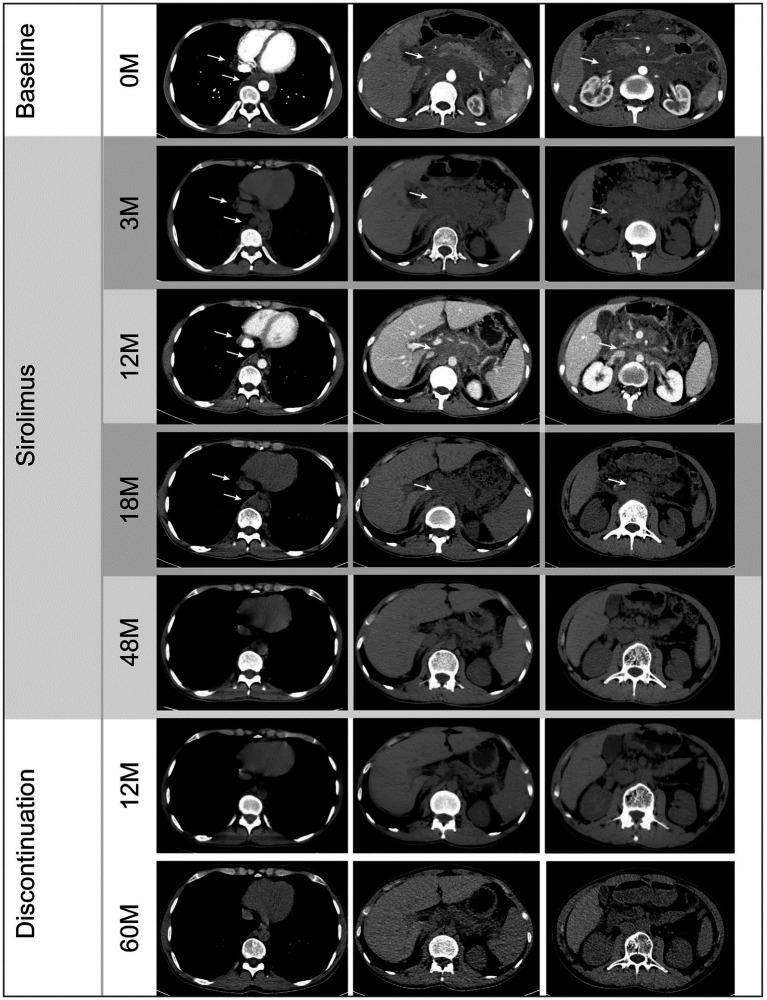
Longitudinal radiologic changes during treatment and follow-up in a patient with kaposiform lymphangiomatosis (KLA). Imaging timepoints include baseline (prior to treatment), sirolimus treatment (Tx) at 3, 12, 18, and 48 months, and post-treatment follow-up (D/C) at 12 and 60 months. “M” denotes months. Baseline CT revealed diffuse soft tissue infiltration in the mediastinum, retroperitoneum, and para-aortic regions. Arrows indicate representative lesions at each timepoint. Lesions gradually regressed during sirolimus treatment (3–48 M) and remained stable after discontinuation (12–60 M), indicating sustained disease control.
